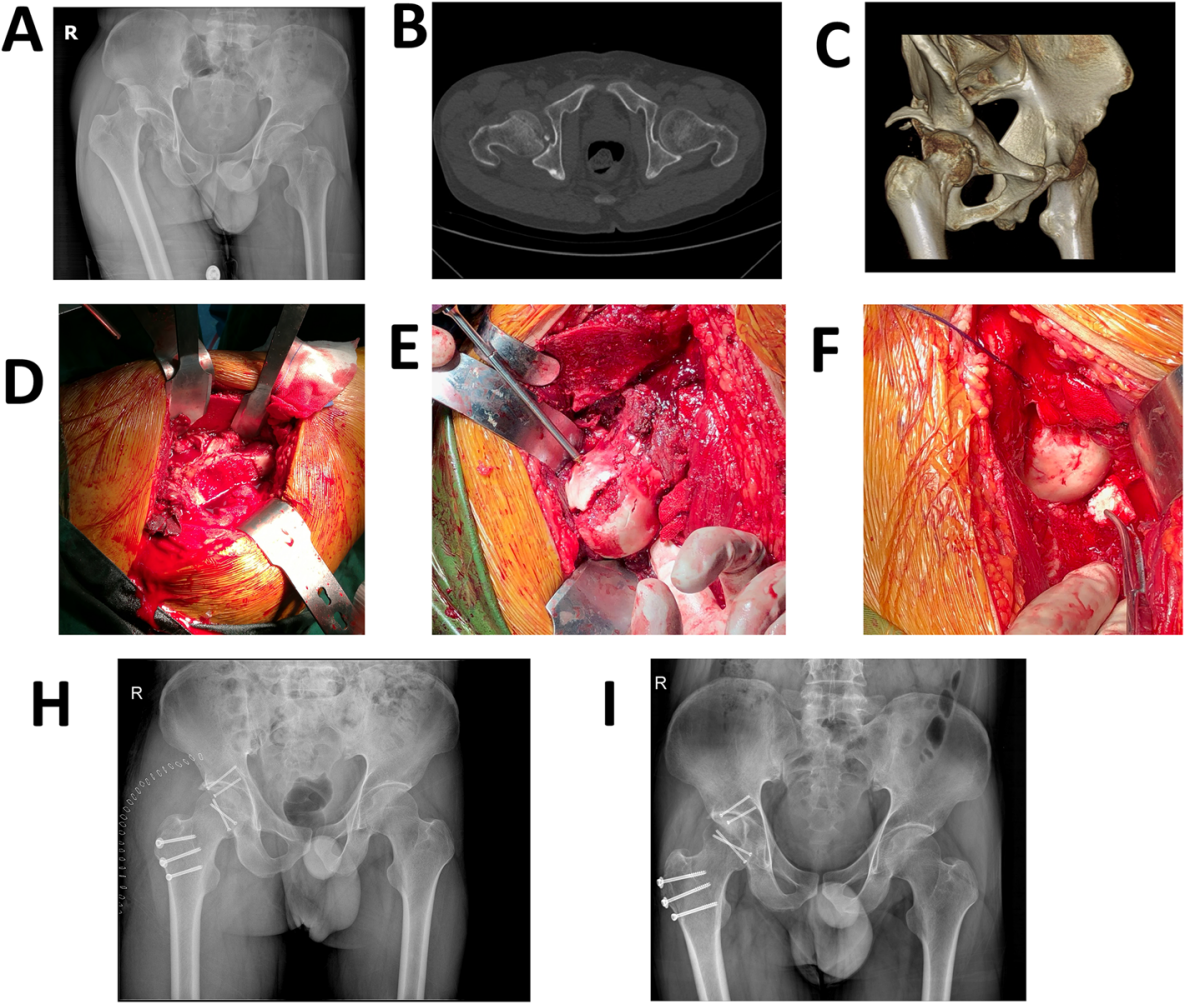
This was a 58 years old male with type IV fracture caused by car accident. a The femoral head fracture, posterior dislocation of the hip, and posterior acetabular wall fracture. b, c The CT scan after reduction which showed detail of the fracture. d The osteotomy of the trochanter: the osteotomized trochanter fragment is reflected anteriorly. e, f The reduction and fixation with femoral head fracture and acetabular fracture. h The X-ray after operation. i The X-ray of 1 year after operation, which showed the fracture has healed and there is no AVN
